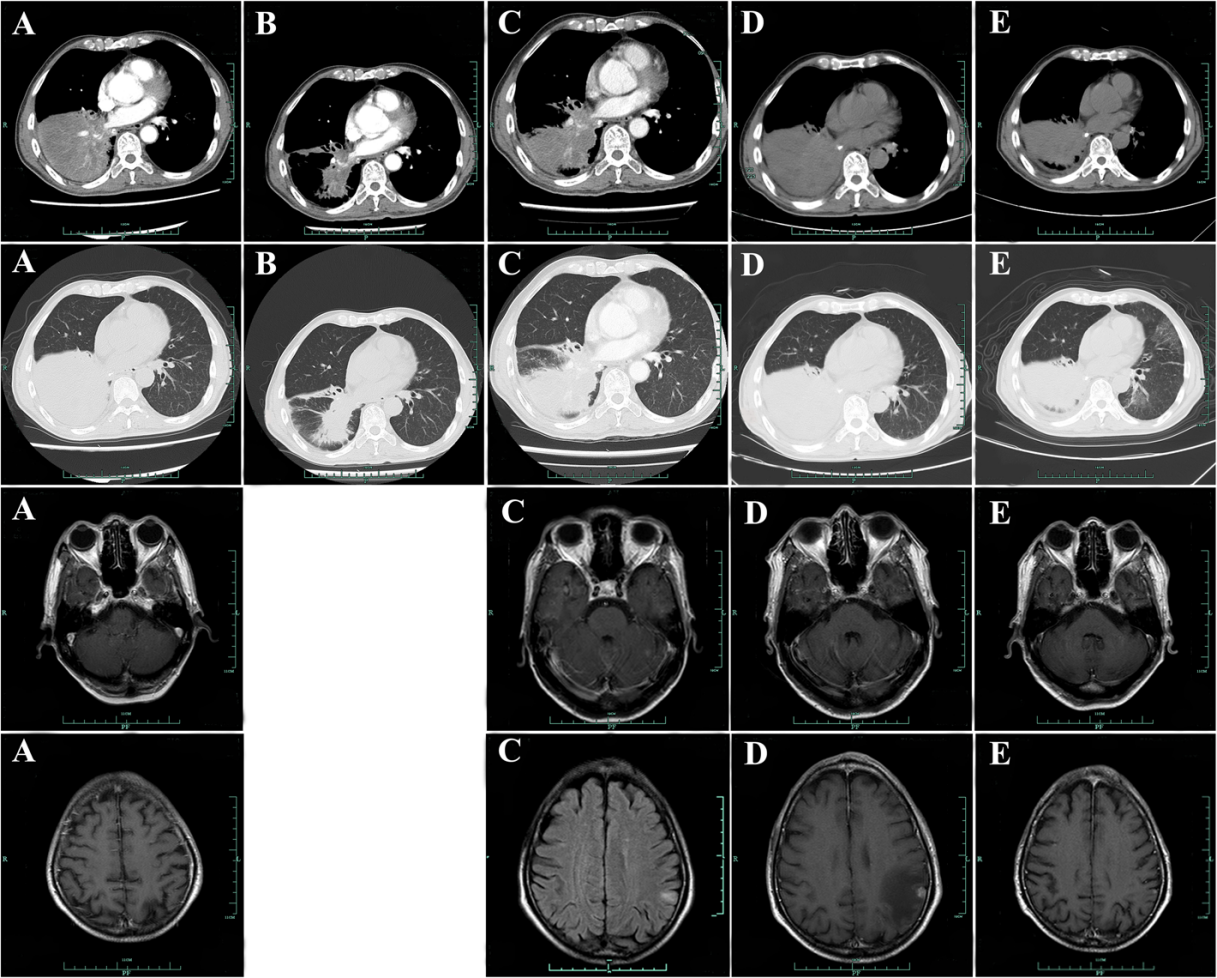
Chest CT scan and brain MRI from May 2017 to February 2018. a Chest CT scan and brain MRI after 2 months treatment of repeating icotinib in May 2017. b Chest CT scan after 3 months treatment of repeating icotinib in June 2017. c Chest CT scan and brain MRI after 6 months treatment of repeating icotinib in September 2017. d Chest CT scan and brain MRI after 8 months treatment of repeating icotinib in November 2017. e Chest CT scan and brain MRI after 1 month treatment of repeating AZD9291 in February 2018
